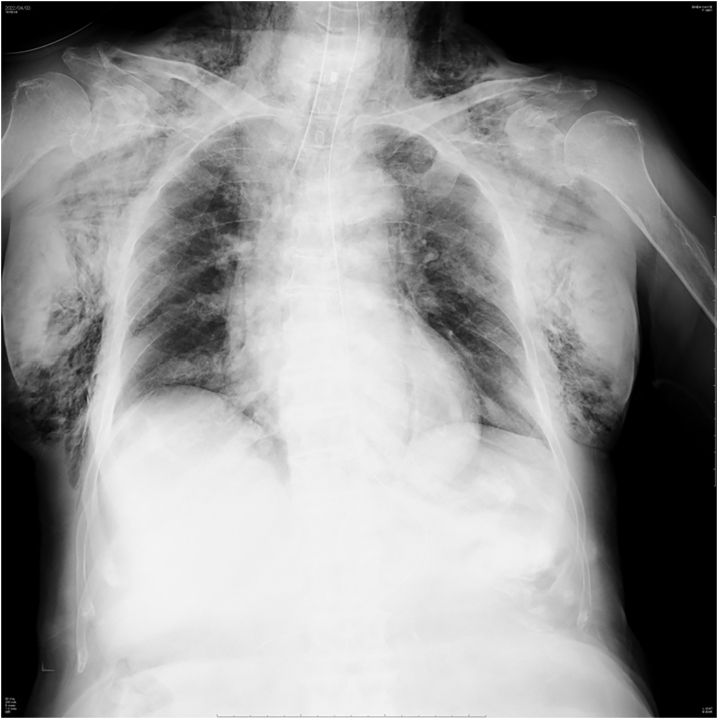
Chest radiograph on admission showing subcutaneous and mediastinal emphysema. Bilateral pneumothorax is unclear.
